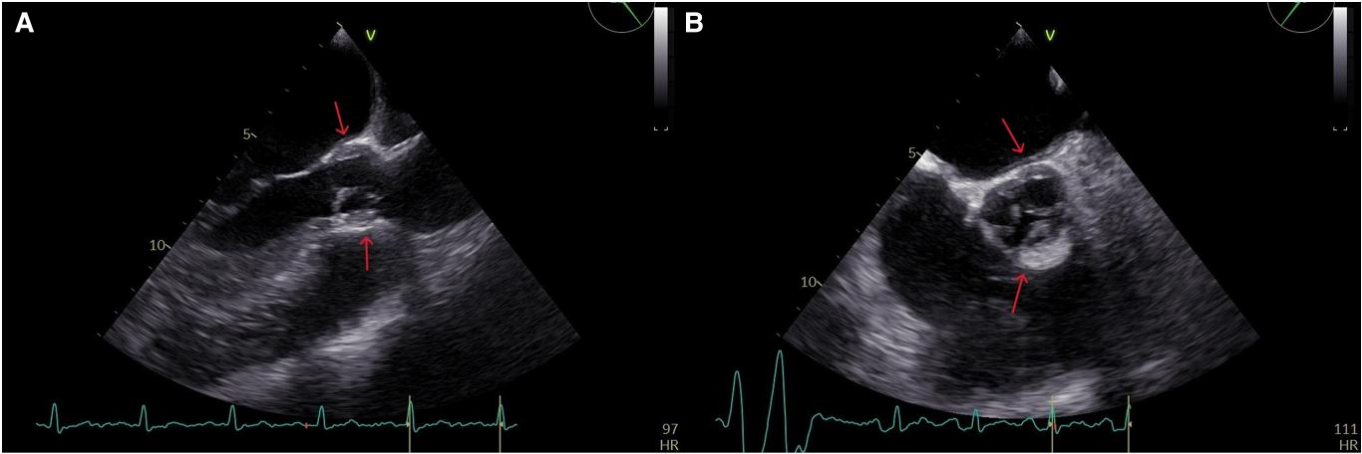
Transesophageal echocardiography in both the three-chamber (*A*) and short-axis views (*B*), showing circular thickening of the aortic root (indicated by the red arrow).
